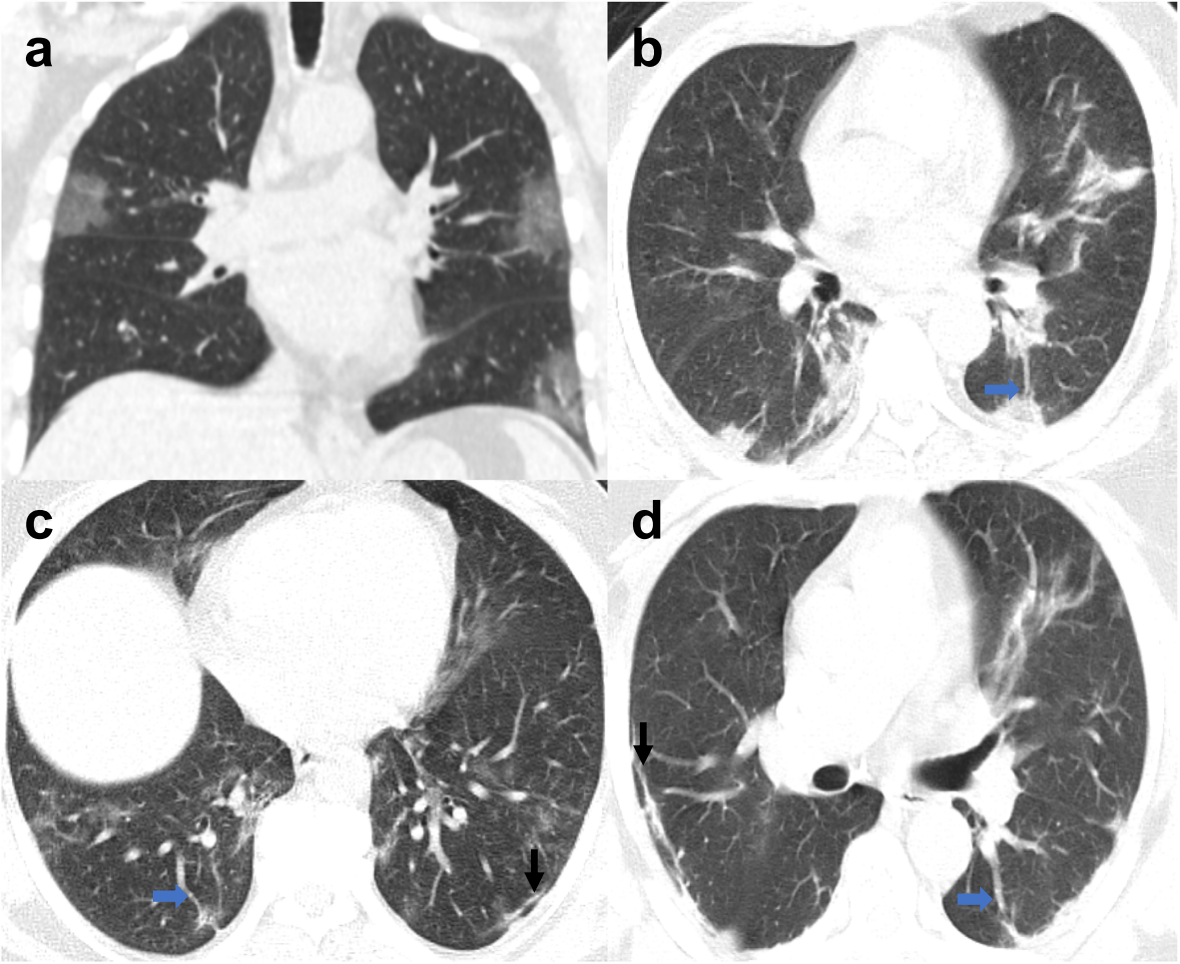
Two-dimensional coronal reconstruction (a) and axial CT image lung window (b, c) in two different cases with COVID-19 pneumonia, showing the typical appearance of bilateral peripheral patchy ground-glass densities (a, c) and/or consolidations (b), subpleural band (black arrows), and vascular dilatation (blue arrows)
